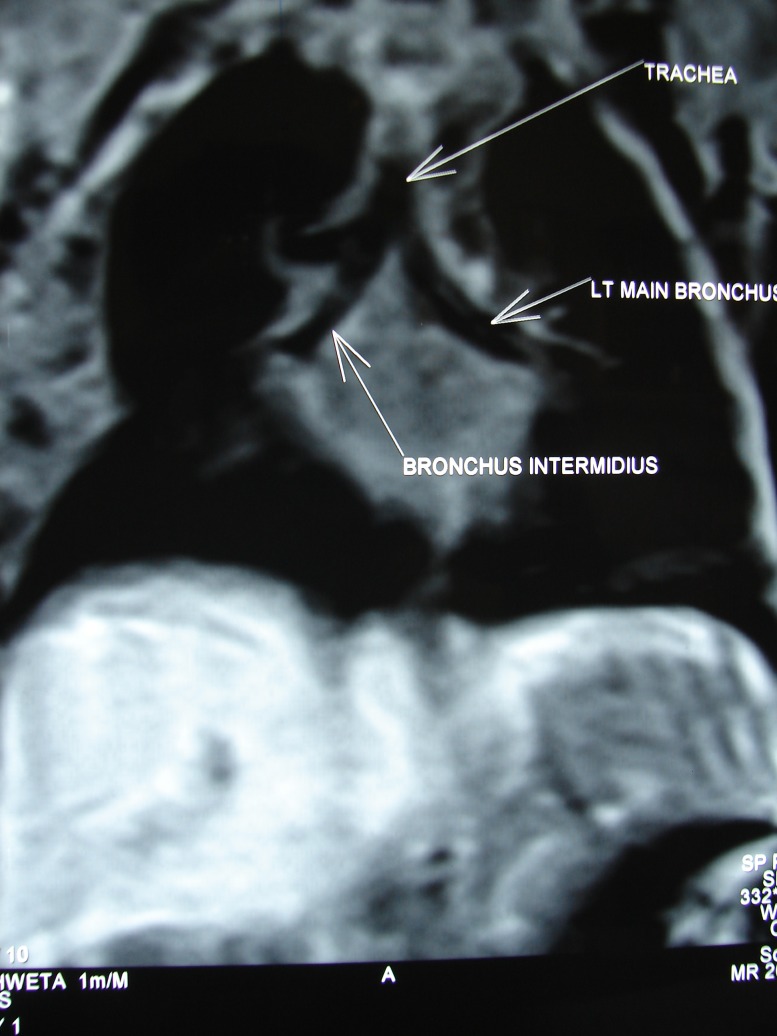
Figure 3: Post-operative MRI showing right upper lobe bronchus originating at the level of the carina.
